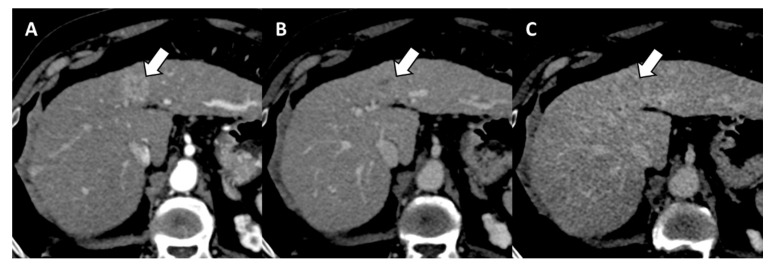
71-year-old woman with cirrhosis who achieved sustained virologic response with direct acting antiviral therapy and history of HCC before HCV eradication. Contrast-enhanced CT reveals a 1.7 cm observation (arrow) nonrim arterial phase hyperenhancement (A), and nonperipheral washout (arrows) on portal venous (B) and delayed (C) phases, consistent with de novo hepatocellular carcinoma at 16 months after DAA.
